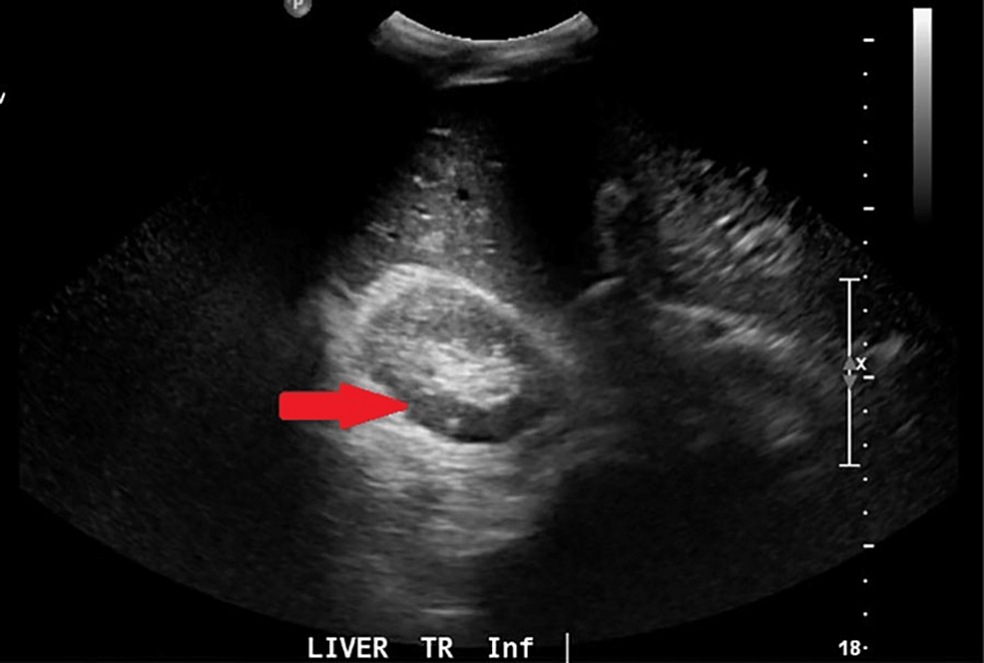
Ultrasound of the liver pointing to portal vein thrombosis.
